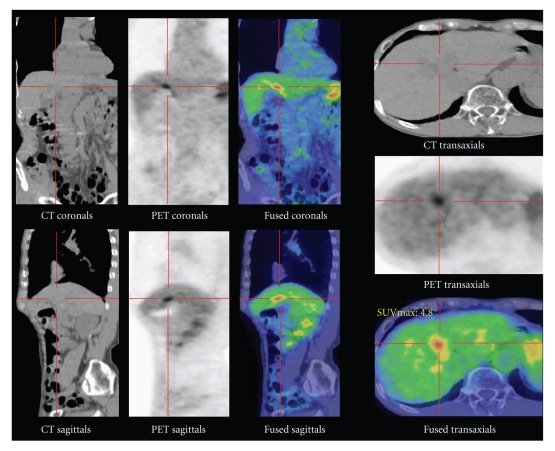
By PET-CT abnormal accumulation of F18-FDP at location considered to be accessory gallbladder could be visualized.
